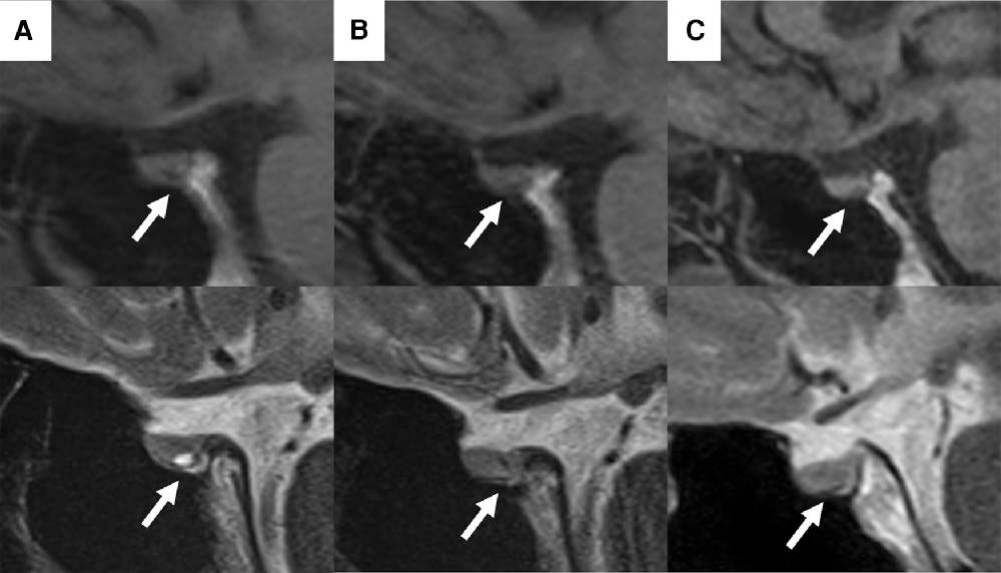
Sagittal T1-weighted (upper) and T2-weighted (lower) MRI scans of the pituitary gland. (A) At the time of referral. A small cystic lesion is visible in the anterior pituitary gland (arrow). (B) Three years after referral. The cystic lesion in the anterior pituitary gland has decreased in size (arrow). (C) Nine years after referral. The small cystic lesion in the anterior pituitary gland remains reduced in size (arrow).
